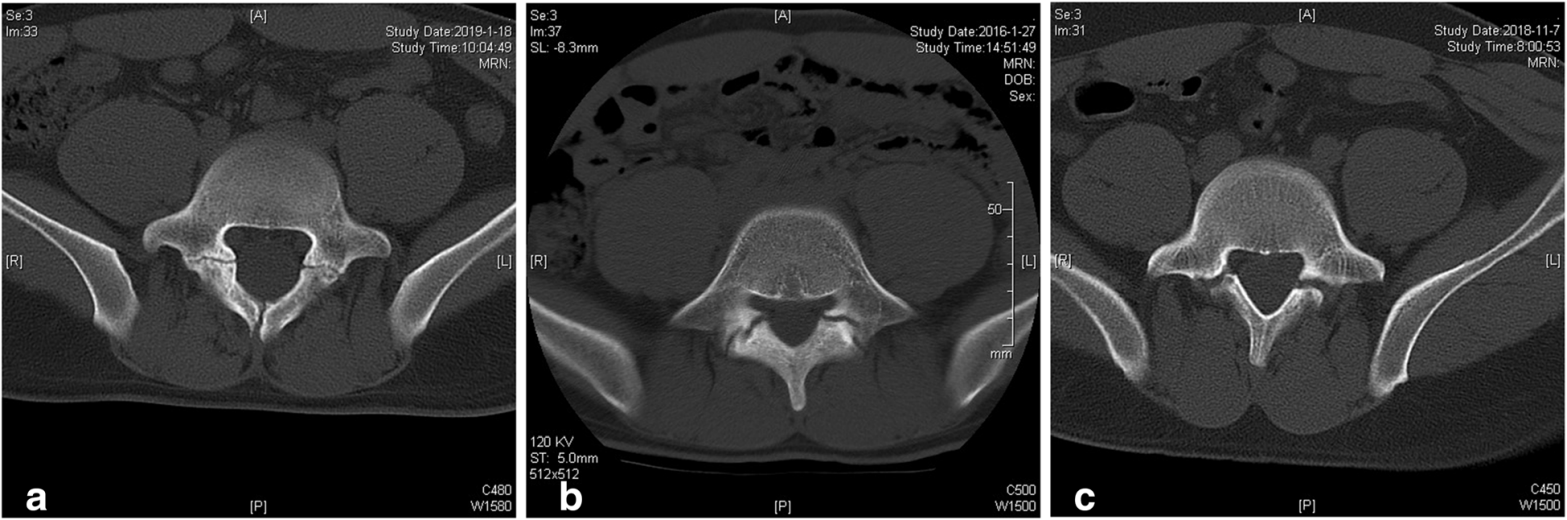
CT classification of lumbar spondylolysis. a Line type, b intermediate type, and c sclerosis type
